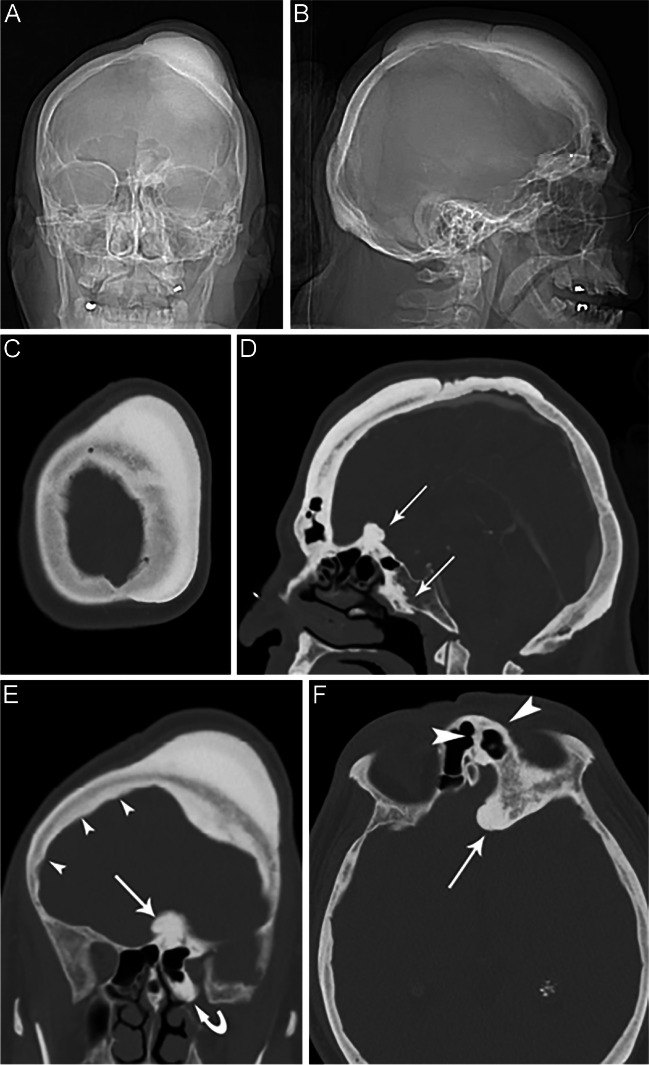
CT angiogram of the head, performed for preoperative planning. Scout images: AP view (A) and lateral view (B). Hyperostotic bone involves the left calvarium in the frontal and parietal region, mainly superficial to the outer cortex. C Axial image showing a thick rind of cortical bone layering on the outer cortex of the left parietal bone. D Sagittal image demonstrating the marked cortical thickening along the frontal bone and involving the sphenoid bone, extending inferiorly along the anterior clivus (arrows). E Coronal image shows the extension of cortical thickening deep to the inner cortex, as well as the involvement of the sphenoid bone and extending distally along the left fovea ethmoidalis and planum sphenoidale. There was marked cortical thickening of the inner table extending into the right frontal bone (arrowheads) and a pedunculated hyperostotic lesion along the planum sphenoidale (arrow). F Axial image at the level of the orbits shows the sphenoid bone involvement and cortical thickening of the left frontal sinus (arrowheads) and the pedunculated lesion along the planum sphenoidale (arrow)
